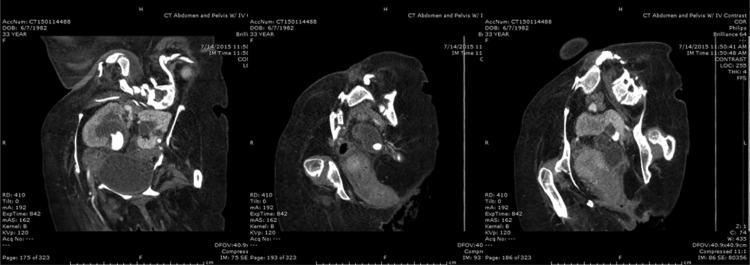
Selected coronal CT images displaying stone burden. From *left* to *right*: right UPJ stone (note curvature of spine); left ureteral stone; left renal stone.
